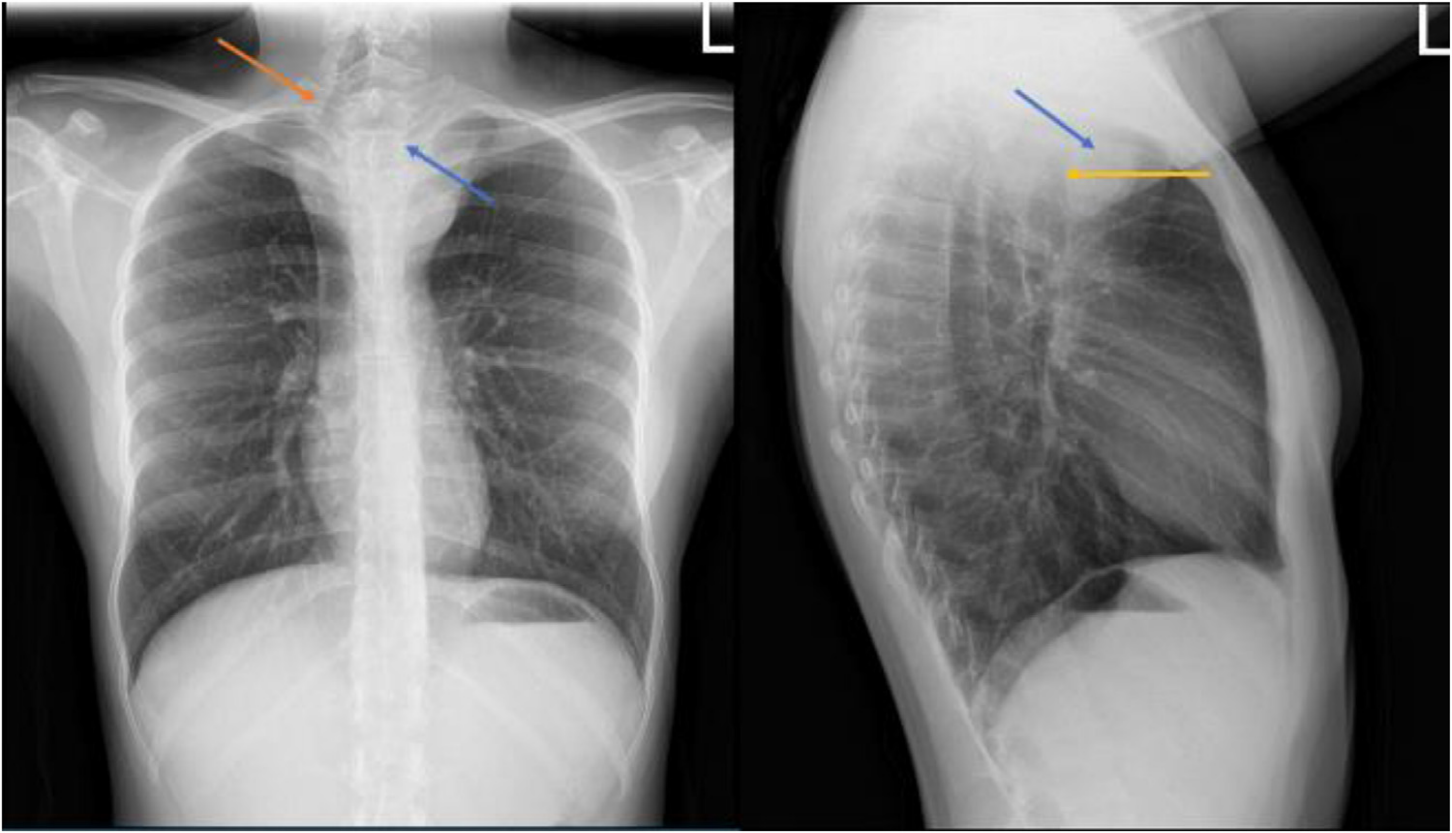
Upright frontal and lateral radiographs demonstrate soft tissue mass (blue arrow) projecting over the mediastinum causing left to right shift of the trachea (orange arrow) with focal stenosis (yellow arrow). (Color version of figure is available online.)
